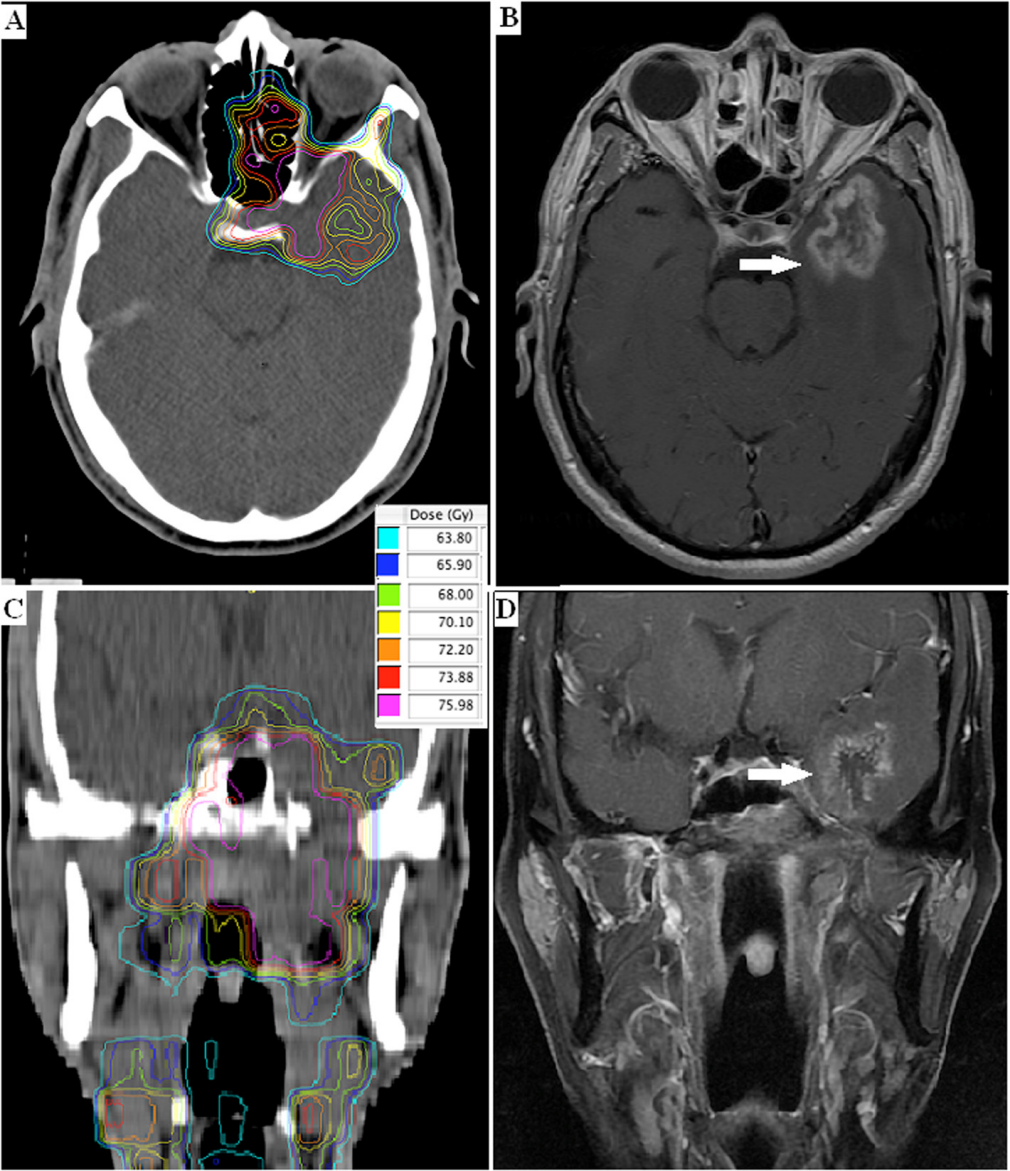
Dose distribution and corresponding necrosis nidus within the temporal lobes (arrow). Axial (A and B) and coronal (C and D) MRI images of a 62-yr-old NPC patient (patient 1 in Table 1).
